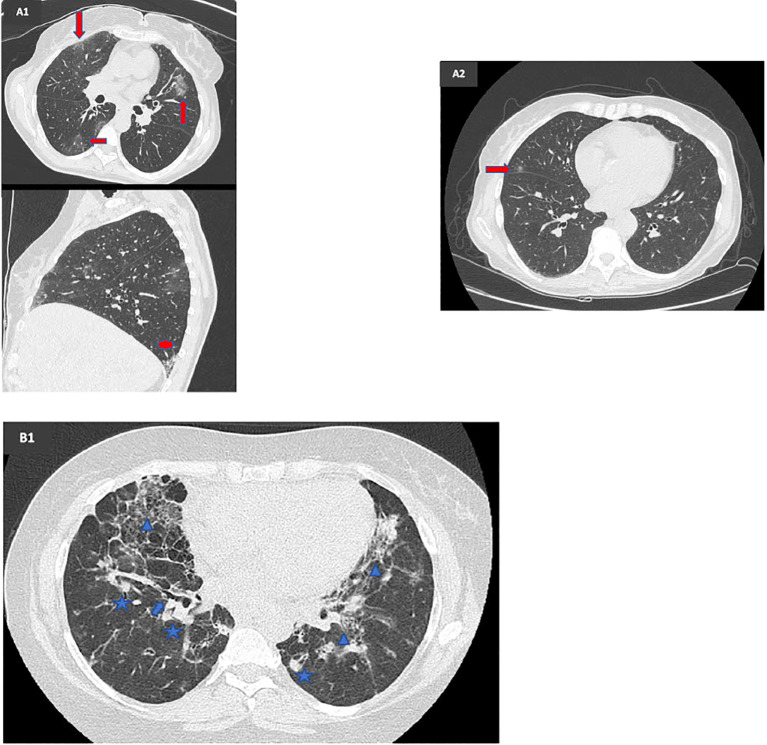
Radiological comparison before and after mycophenolate mofetil (MMF) therapy in two patients with a CVID-related GLILD complicated by SARS-CoV-2 infection (A1) Lung HRCT executed in February 2021, during a GLILD flare, prior to MMF therapy, showing multiple dense nodular areas (arrowhead) bilaterally with modest thickening of the interstitial septa (star) and ground-glass areas (arrow) especially in the left side. (A2) Lung HRCT executed in March 2022, 10 months after MMF start, showing a sharp radiological improvement with resolution of ground-glass areas. (B1) Lung HRCT executed in June 2020, prior to MMF therapy, showing multiple ground-glass (arrowhead) and dense nodular areas (star) distributed bilaterally, with interstitial reticular thickening and bronchiectasis foci (arrow).
